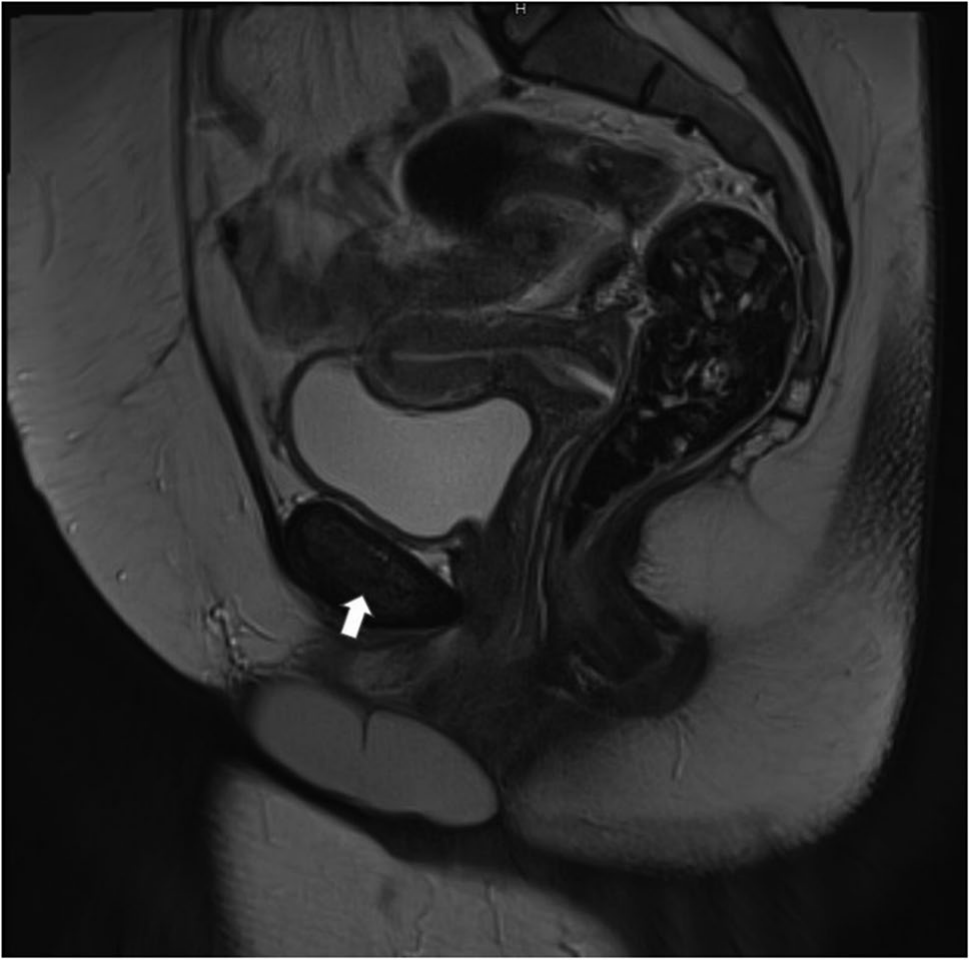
MRI sagittal view of clitoral cyst
